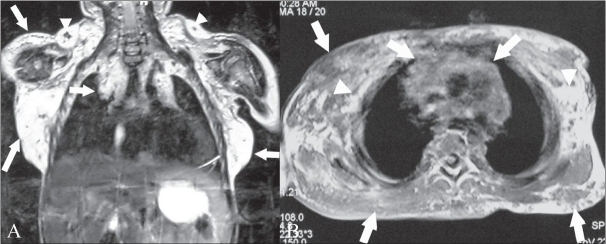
Fat-saturated, coronal T2W MRI image (A) of the chest and abdomen shows a diffusely infiltrating hyperintense lesion (arrows) in the soft tissues of the lower neck, shoulder, axillae, and chest wall, extending into the anterior mediastinum (arrows) with a few areas of focal hypointensity (arrowheads). The axial T1W MRI image (B) of the upper thorax shows the lesion to be hypointense (arrows) with interspersed fat (arrowheads)
